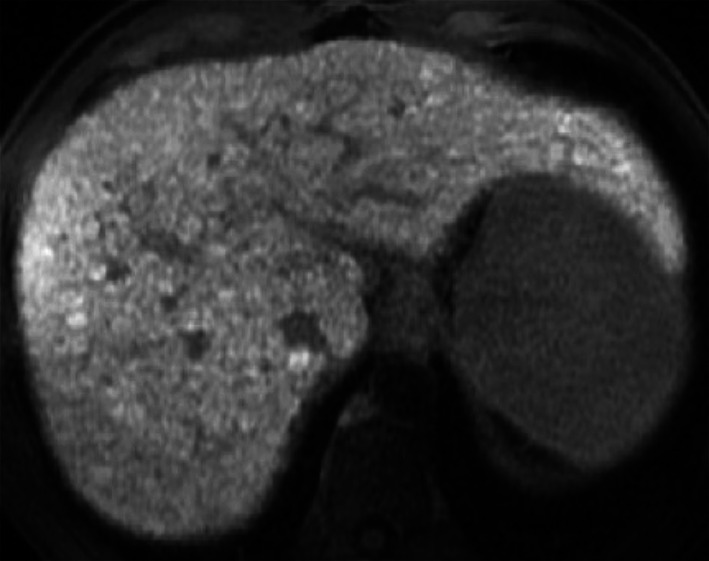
A 43-year-old man with HCV-related cirrhosis and multiple cirrhotic regenerative nodules. Gadoxetate disodium-enhanced MRI shows multiple cirrhotic regenerative nodules that are hyperintense on hepatobiliary phase
